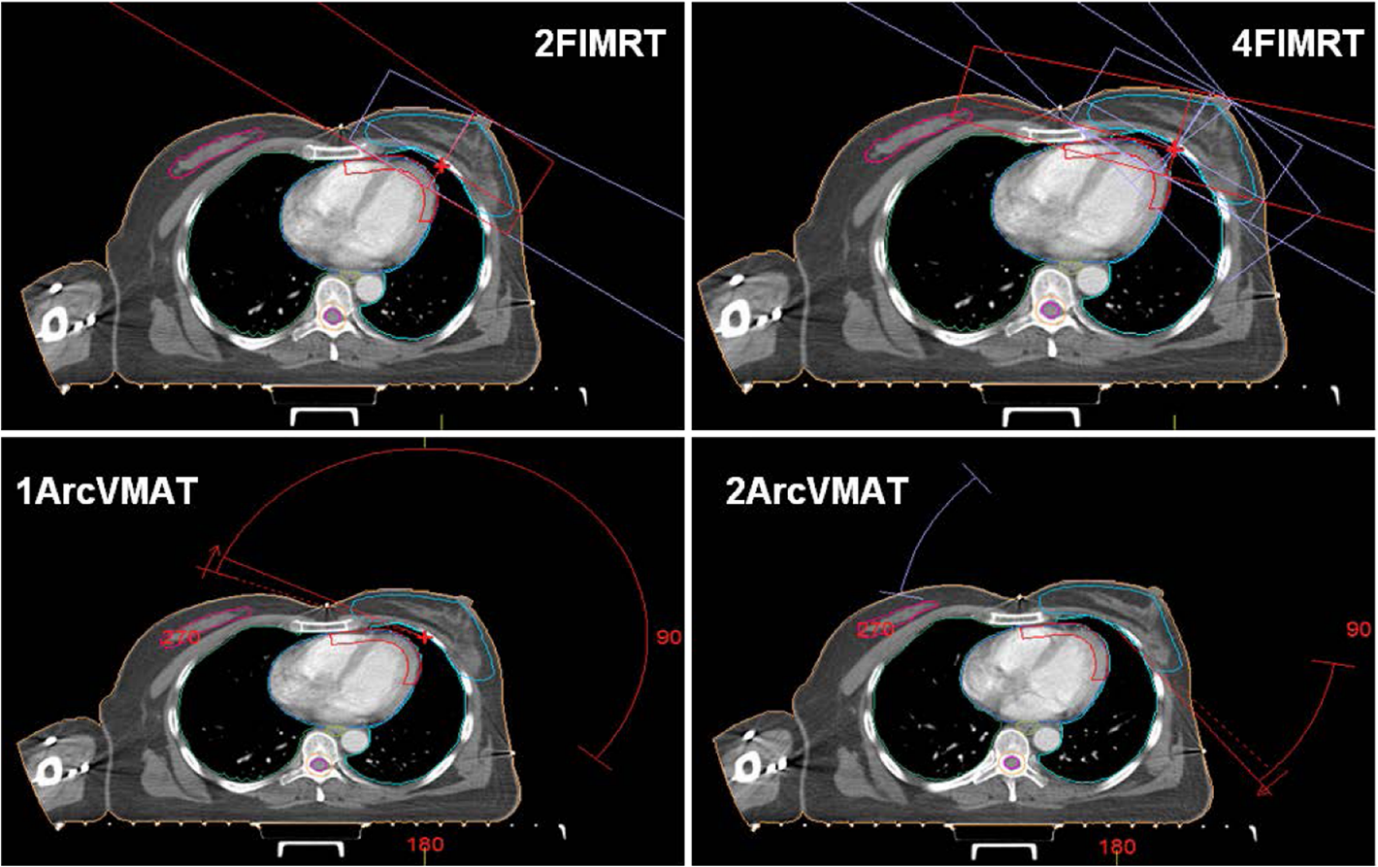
IMRT and VMAT treatment plans with corresponding examples of segment shapes and digitally reconstructed radiographs (DRRs) for left-sided breast cancer
